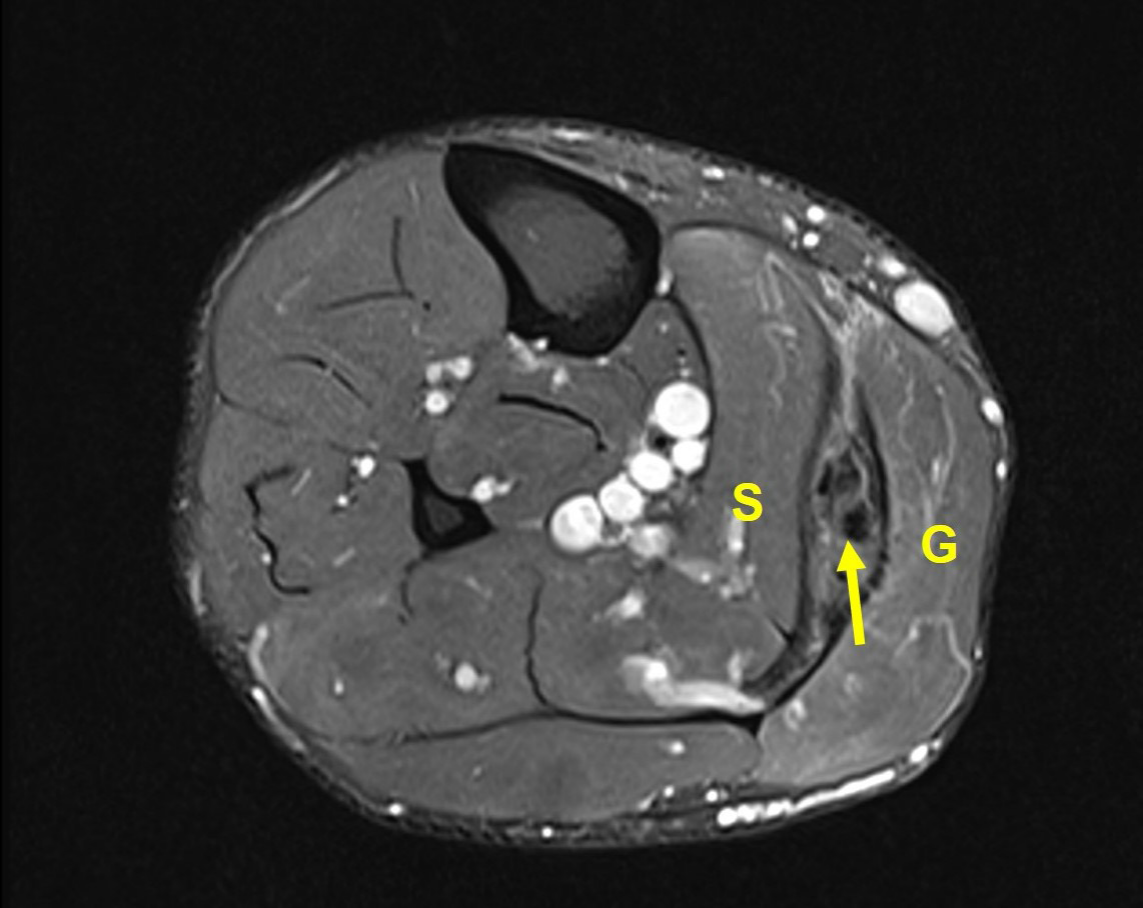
MRI axial section of the right calf showing the location of the pseudotumor (arrow) between the gastrocnemius (G) and soleus (S) muscles.
